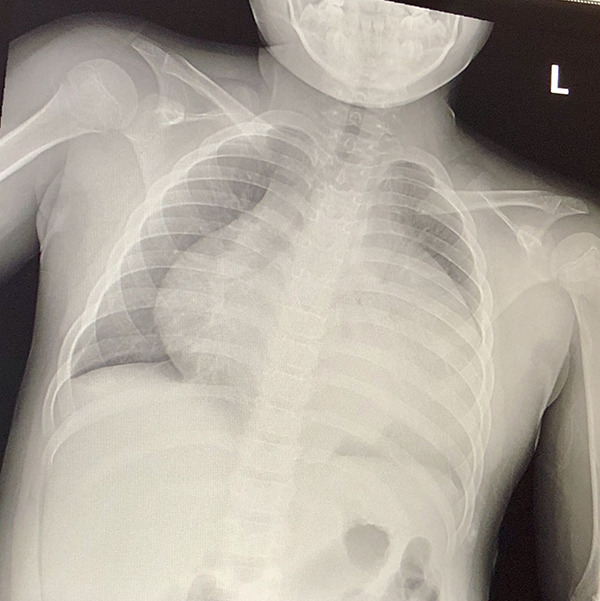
Chest X-ray (CXR) with enlarged cardiac silhouette with bilateral small pleural
